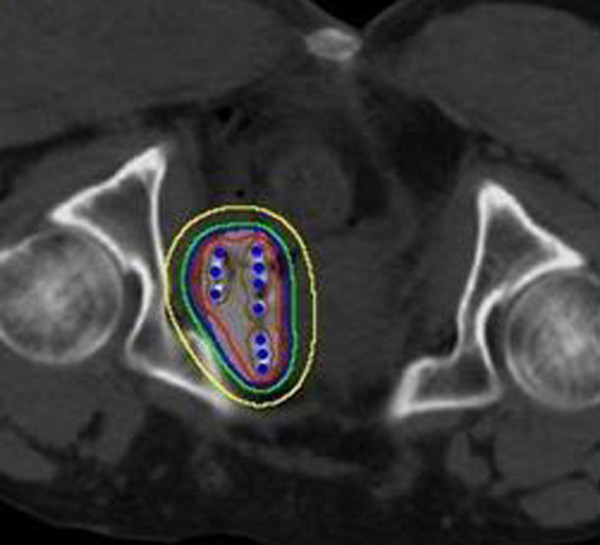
A typical transverse slice showing the distributions of ^125^I seeds and isodose curves after seed implantation from CT scan. The isodose lines shown are 300 Gy (brown), 160 Gy (red), 100 Gy (blue), 80 Gy (green) and 40 Gy (yellow). PTV and ^125^I seeds are shown in light grey circle and blue dots, respectively.
